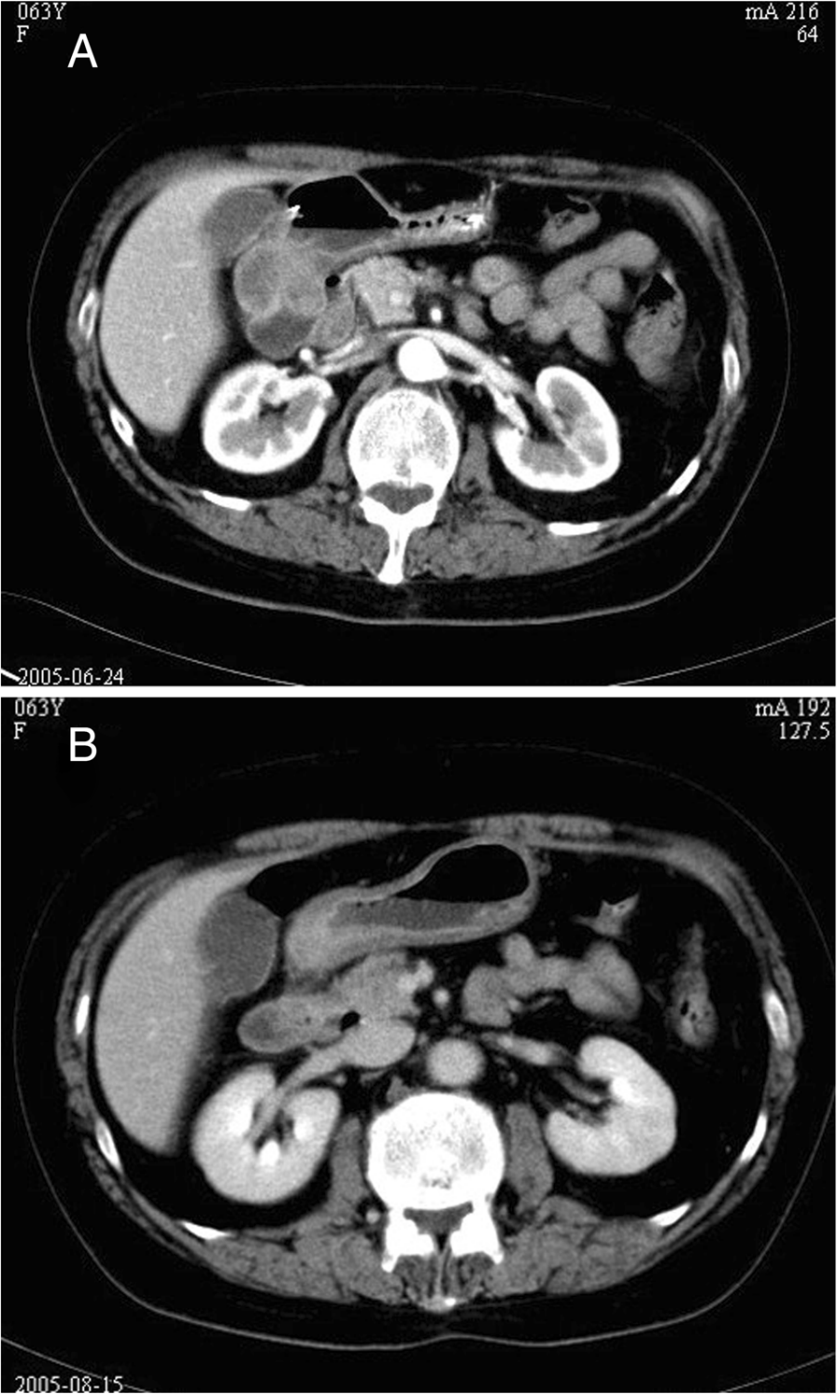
Computed tomography of the gastric wall. (A) Abdominal computed tomography (CT) scans revealed thickening of the gastric wall. Obliteration of the fat planes between the gastric tumor and adjacent organs indicated a T4 tumor. (B) CT scans after two courses of chemotherapy showed dramatic reduction of the primary lesion and clarification of the fat planes.
